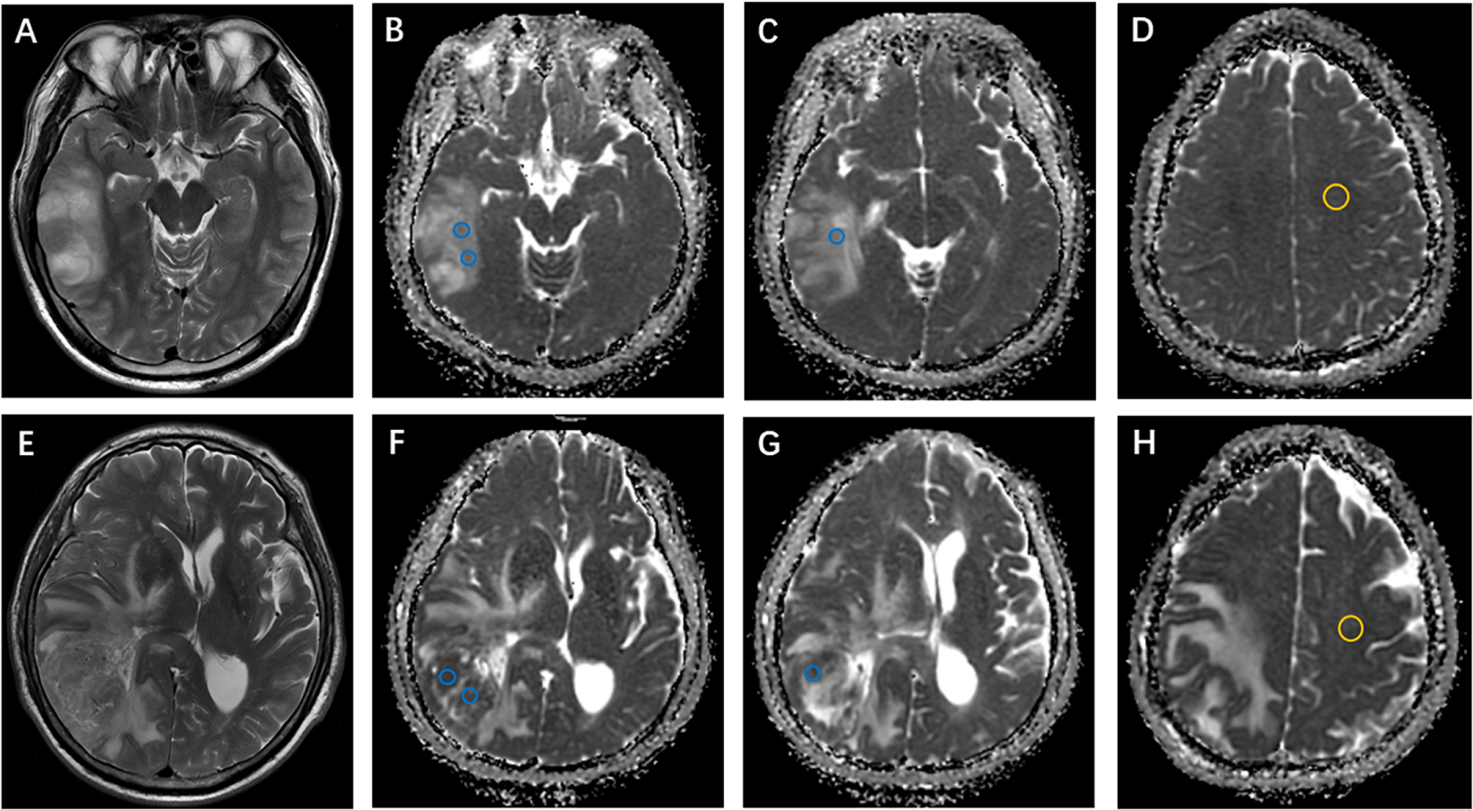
Examples of apparent diffusion coefficient (ADC) measurements. (A–D) Axial T2-weighted imaging shows an isocitrate dehydrogenase (IDH)-mutant oligodendroglioma in the right temporal lobe. ADC maps show the regions of interest used to determine ADCmin (perceived lowest ADC regions, blue) and ADCn (contralateral, normal-appearing white matter, yellow). (E–H) Axial T2-weighted imaging of a right temporal IDH-wildtype glioblastoma. ADCmin and ADCn were calculated using the same method as above.
